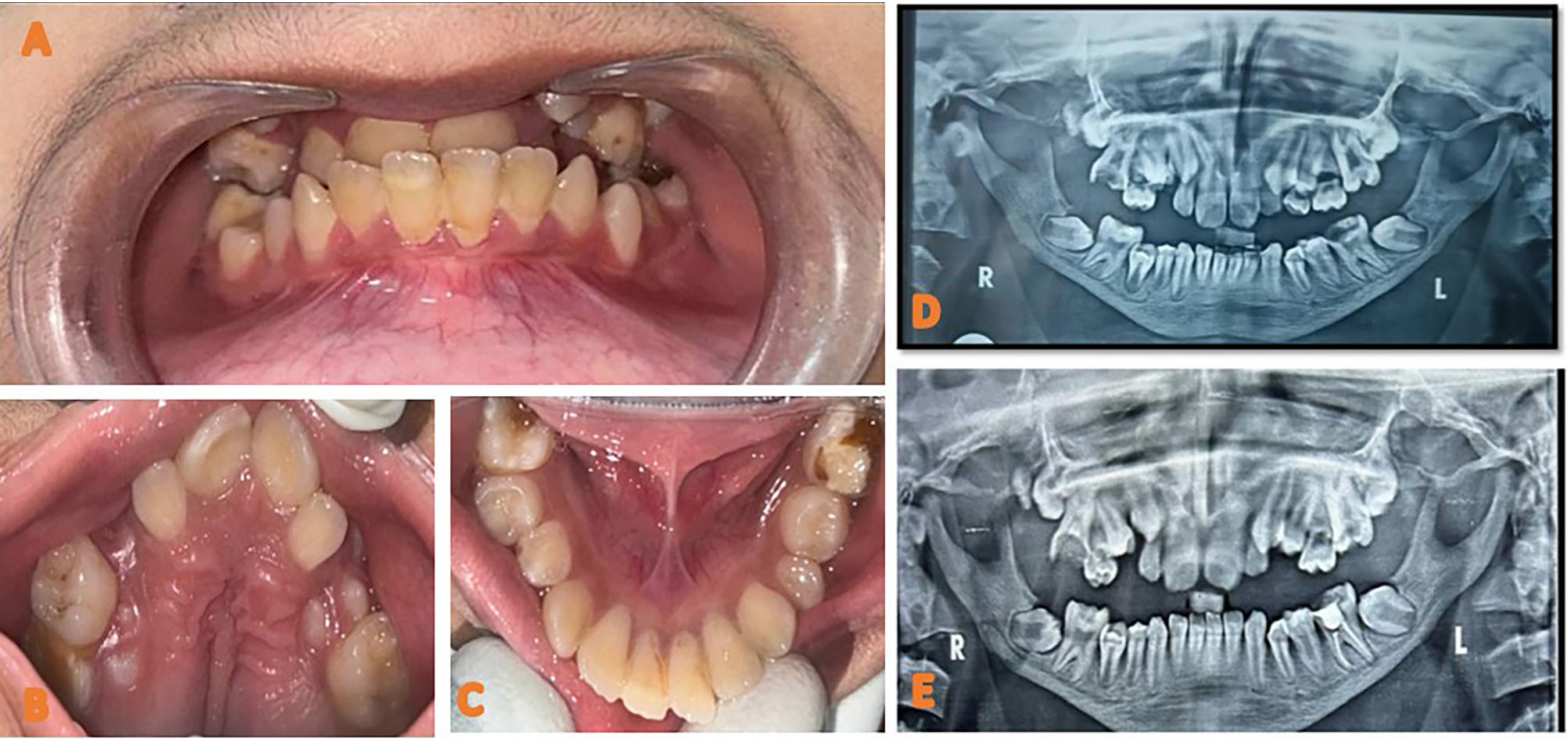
A and E: (A) Intraoral picture depicting anterior and posterior crossbite. (B) Intraoral view of maxilla showing prominent palatal rugae, deep palate, crowded upper anteriors and constricted maxillary arch (C)Intraoral view of mandible showing proclined mandibular incisors, dental caries in relation to left and right lower first permanent molar. (D) Preoperative orthopantomogram showing dental caries in relation to 16 and 36 , hypoplastic jaw, taurodontism impacted permanent teeth and retained deciduous teeth (E) Postoperative orthopantomogram showing Root canal therapy done with respect to 36 and MTA pulpotomy done with respect to 16 followed by restoration with Glass Ionomer Cement.
